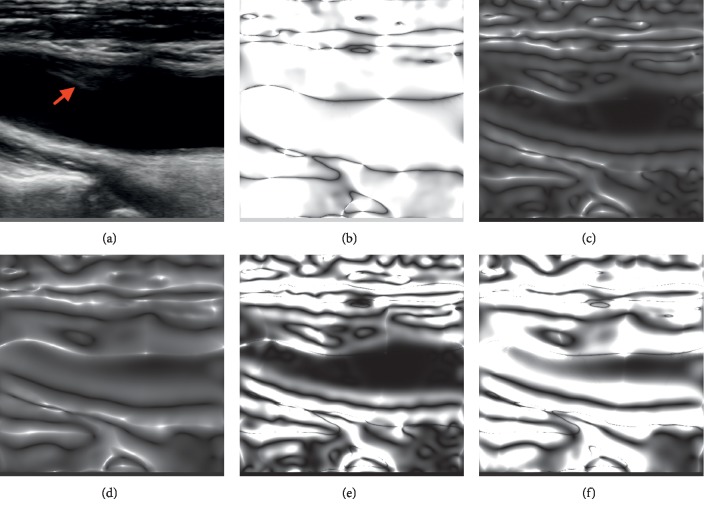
B-mode ultrasound image (a) and its local orientation images (b) and local phase images (c, d). Local orientation image with a confidence coefficient (e, f), with a smaller standard deviation ($\sigma_{1}=10\text{ and }\sigma_{2}=10\sqrt{2}$) (c, e), and with a larger standard deviation ($\sigma_{1}=20\text{ and }\sigma_{2}=20\sqrt{2}$) (d, f).
